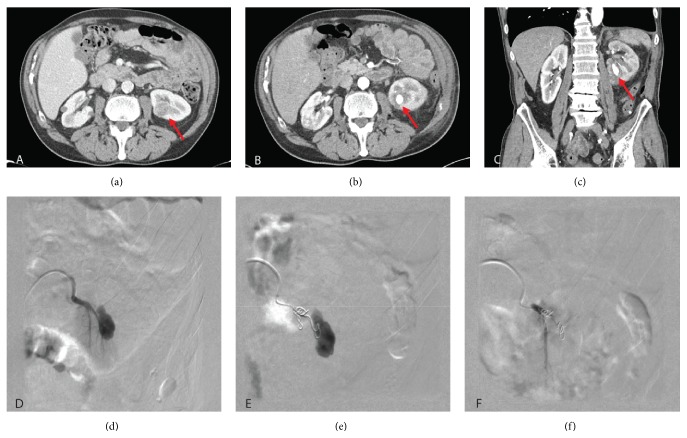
Preoperative CT scan, CTA, and arterial embolization of PRA. (a) Preoperative CT-scan of a central endophytic renal mass (red arrow). ((b), (c)) Transversal and coronary view of a postoperative CT-scan showing a RPA in the former resection area (red arrow). (d) Digital subtraction angiography (DSA) of the lower pole segmental renal artery showing the RPA. (e) Superselective DSA via microcatheter after embolization with 4 microcoils showing remaining perfusion of the RPA. (f) Superselective DSA via microcatheter after another 4 min: total occlusion of the RP with rest perfusion of the lower pole.
